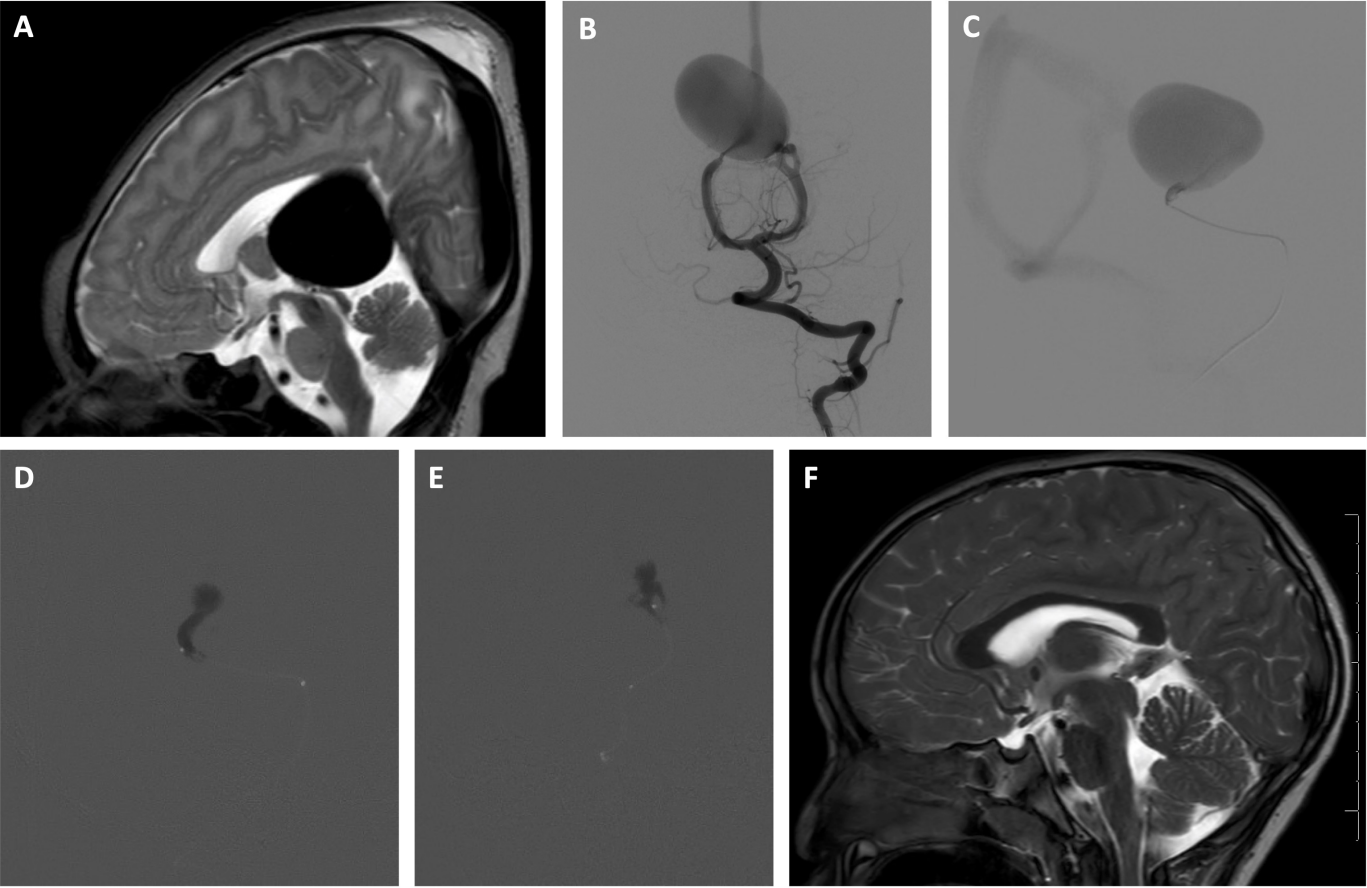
Two-stage embolization of VOGM with n-BCA glue using adjunctive RVOP for flow control. A newborn male, initially found to have a VOGM on prenatal ultrasound performed late in pregnancy because of maternal risk factors was asymptomatic and monitored closely during the first 3 months of life. Use of the RVOP technique was selected for this case given the anticipated rapid transit of embolysate through the hight-flow shunt. A transvenous approach was not selected due to the anticipated challenge of navigating a microcatheter through the profoundly dilated venous pouch. A transarterial balloon-assisted embolization was not selected due to multiple high-flow arterial inputs that could continue to propel the embolysate downstream despite balloon inflation. Utilizing the RVOP adjunctive technique, a two-stage transarterial acrylic embolization with adjunctive right ventricular overdrive pacing was performed resulting in angiographic cure. At three years follow up, the patient is neurologically normal and attending preschool with normal development. (A) Sagittal T2 weighted MR image of the brain obtained on day of life 1 shows massively dilated aneurysmal median prosencephalic vein, consistent with vein of Galen malformation. (B) Early arterial phase of selective left vertebral artery angiogram in frontal projection just prior to first stage embolization at 3 months of age shows multiple, bilateral mural arteriovenous macrofistulae formed between medial posterior choroidal arteries and aneurysmally dilated median prosencephalic vein (MPV). (C) Microcatheter angiogram (lateral projection) of mural arteriovenous shunt supplied by left medial posterior choroidal artery performed through Echelon 10® microcatheter (Medtronic, Santa Rosa, CA) during first stage embolization shows rapid arteriovenous shunting. The microcatheter is stationed just proximal to a sharp bend in the microcatheter bearing artery to reduce the possibility of venous escape. (D,E) Lateral (D) and frontal (E) projections of acrylic cast in the mural arteriovenous shunts supplied by left medial posterior choroidal artery after stage 1 embolization. The embolization was performed during right ventricular overdrive pacing with a 4 French bipolar pacing catheter, pacing at 250 beats per minute for 20 s. Embolization was performed with a mixture of 75% n-BCA and 25% Ethiodol through an Echelon 10® microcatheter. Venous escape of embolic agent was completely suppressed without procedure related complications. Sudden conversion to sluggish flow within the aneurysmal vein (Medtronic, Santa Rosa, CA) pouch prompted anticoagulation for two weeks. Second stage embolization of the mural arteriovenous shunt supplied by the right medial posterior choroidal artery was performed in identical fashion two weeks later without venous escape or other procedure related complications. Complete cerebral angiography performed 3 months after second stage embolization confirmed complete angiographic occlusion of the VOGM. (F) At 3 years follow up, sagittal T2 weighted MR image of the brain shows complete involution of the aneurysmal median prosencephalic vein.
